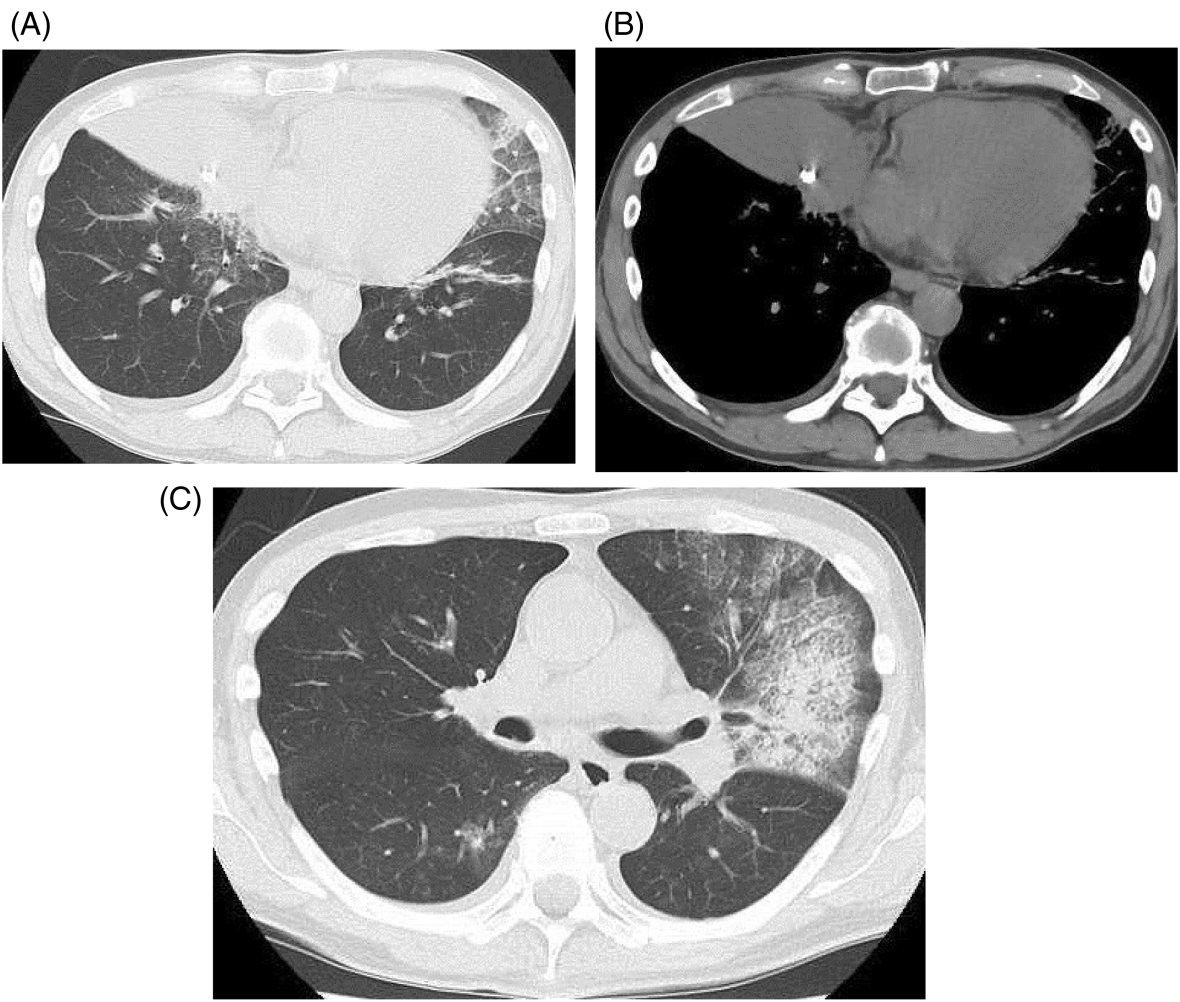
Chest computed tomography at the first visit shows right middle lobe atelectasis, an artefact stuck in the right middle lobe bronchus (A and B), and an infiltration shadow in the lingular segment of the left lung (C).
